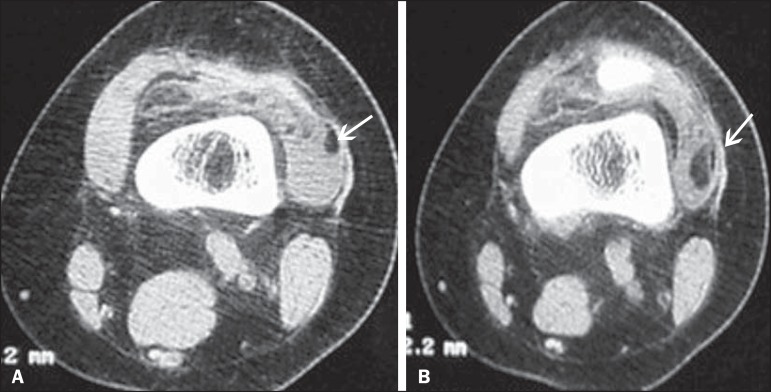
Lipoma arborescens. CT scans, both acquired in the axial plane. The
